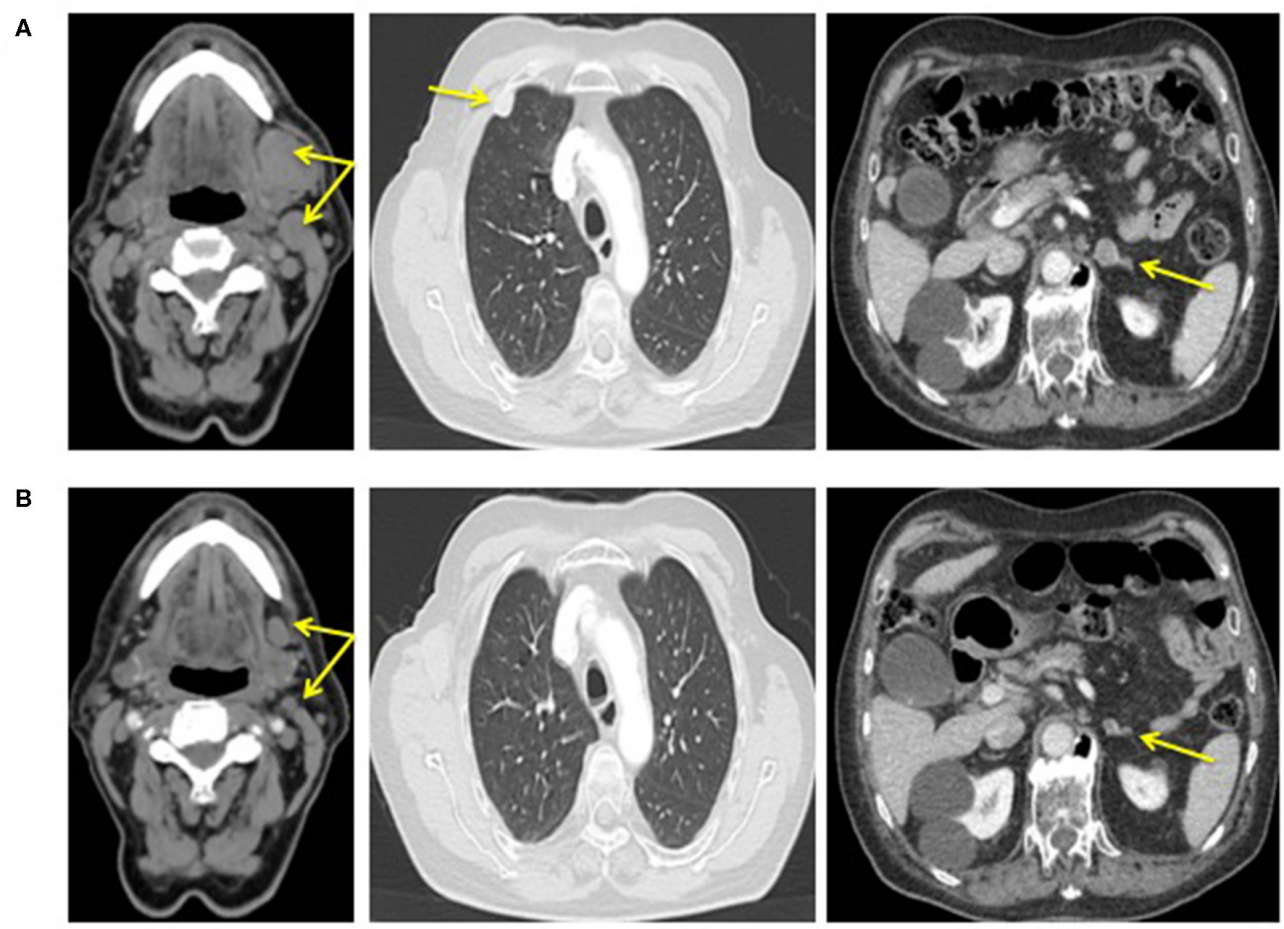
CT scan performed before therapy with the association octreotide plus avelumab (A) and after 10 months of therapy (B).
